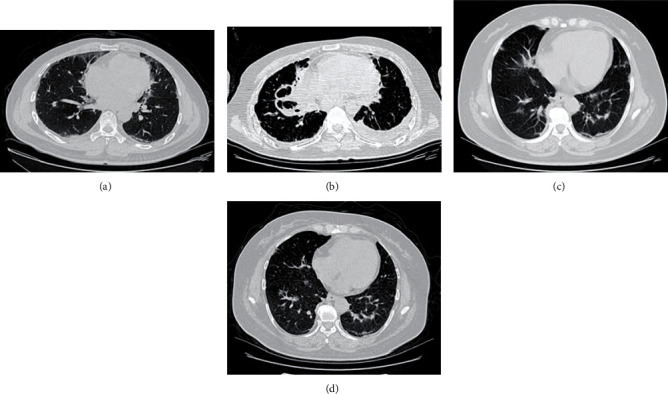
(a, b) CT images of paraquat poisoning in a 53-year-old man at the beginning and 2 months later. (c, d) CT images of paraquat poisoning in a 40-year-old woman at the beginning and 2 months later.
